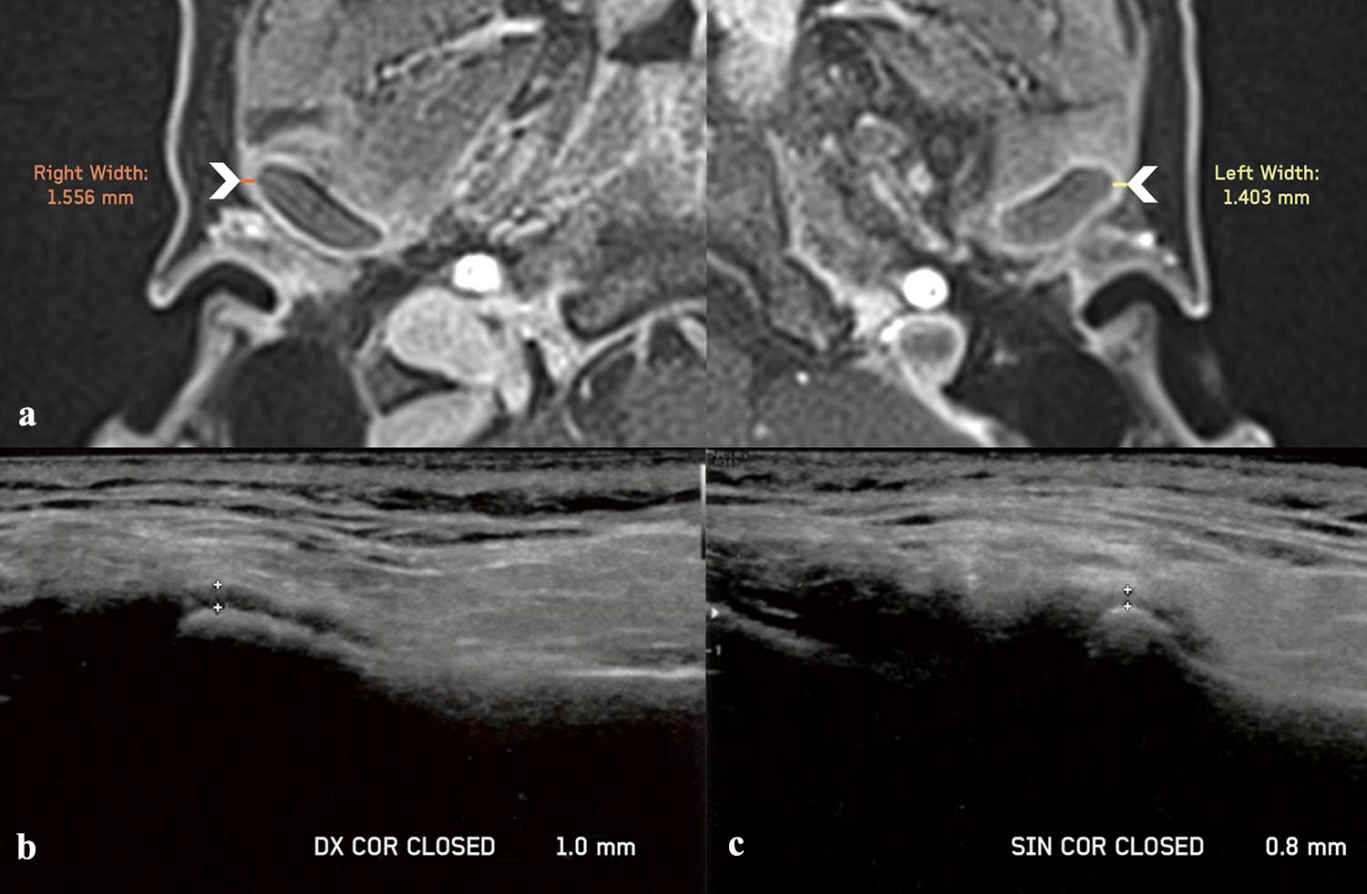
Lateral periarticular space (LPAS) assessment with MRI and US in a patient with JIA and active involvement of both TMJs. a Axial postcontrast MRI images of the right and the left TMJs show periarticular enhancement, with an LPAS width of 1.556 mm and 1.403 mm, respectively (arrowheads). b, c US images of the right and left TMJs show an LPAS width of 1 mm and 0.8 mm, respectively
